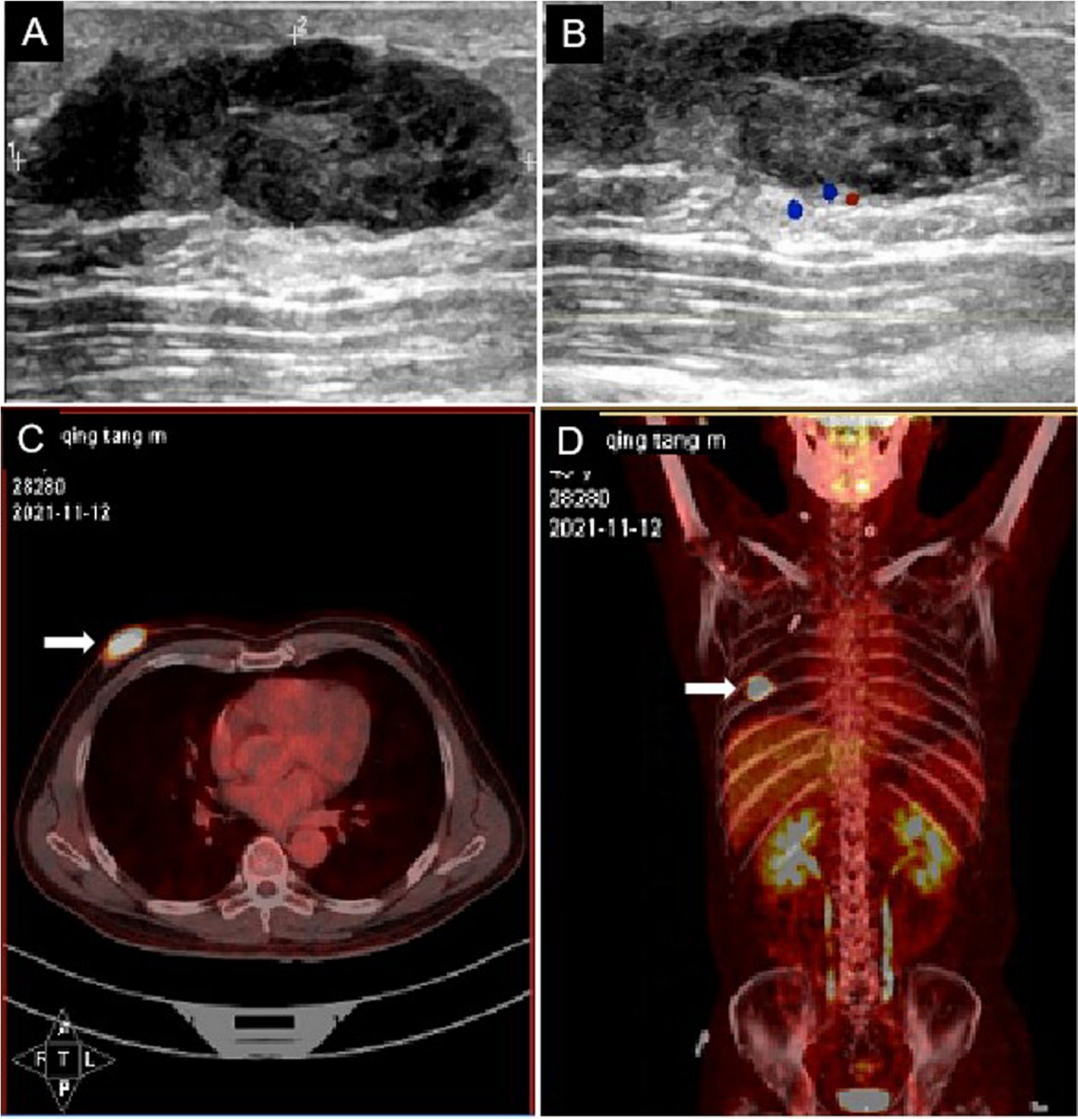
Imaging of primary breast diffuse large B cell lymphoma in a male patient. B-ultrasound of the breast revealed a low-echo mass behind the right nipple with clear boundaries, irregular shape, and uneven internal echo (A). Color Doppler flow imaging (CDFI) showed a blood flow signal around the mass (B, star). Systemic positron emission tomography/computed tomography (PET/CT) showed abnormally elevated dextrolactose metabolism (C and D, arrow), which was considered a malignant lesion
